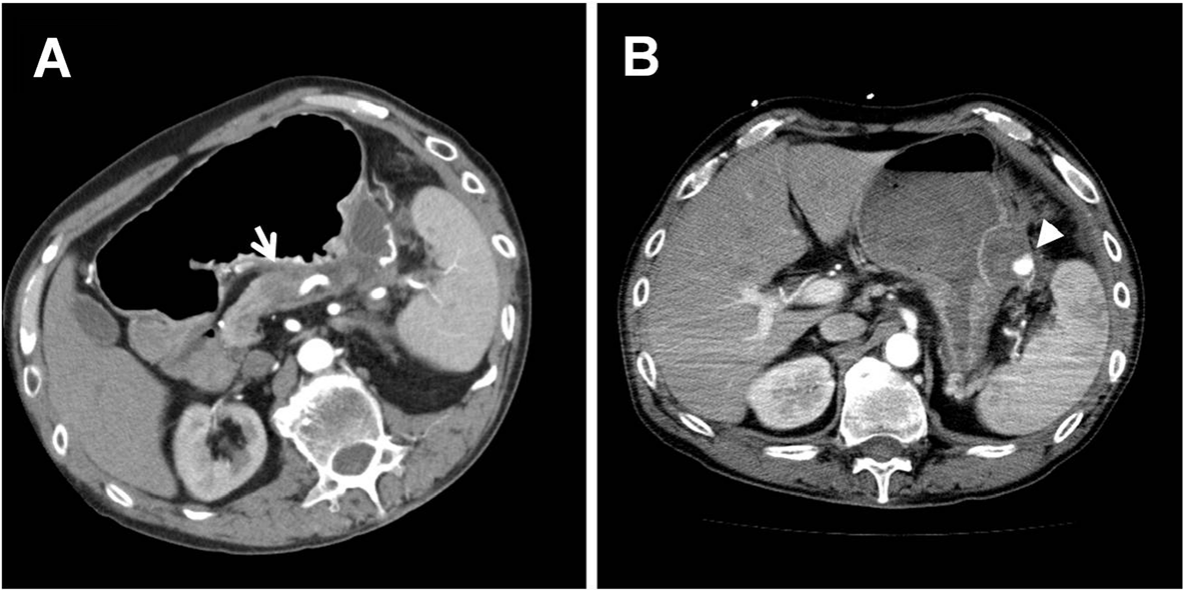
Abdominal computed tomography (CT) of the patient. a An initial abdominal CT revealed a low-density mass in the pancreatic body (*arrow*) and a cystic lesion in the pancreatic tail. b One month later, a pseudoaneurysm in close contact with the wall of pancreatic pseudocyst was clearly visualized (*arrow head*)
